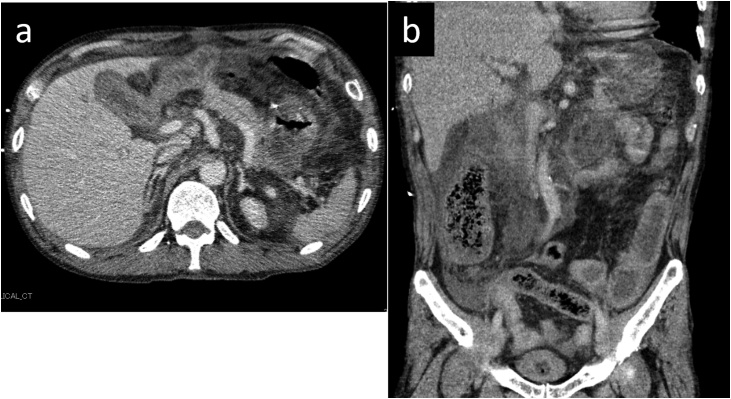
Contrast-enhanced CT scan showing spread of inflammation throughout the abdomen. (a) Axial view, (b) Coronal view.
